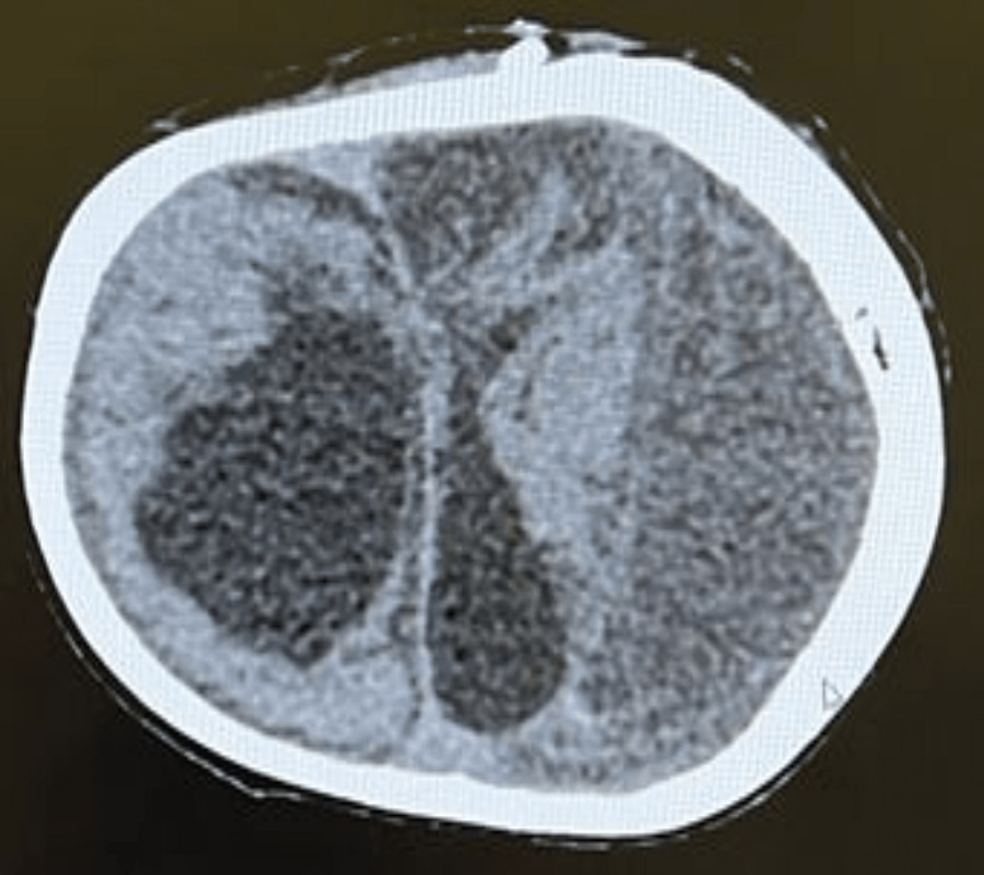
Repeated brain CT. The image reveals that the tip of the VP shunt is situated in the encysted hydrocephalus on the right side. It also indicates an interval decrease in the size of the supratentorial hydrocephalus. Additionally, the image shows an interval increase in the size of the left cerebral convexity subdural collection, along with the development of a small right cerebral convexity subdural collection.
